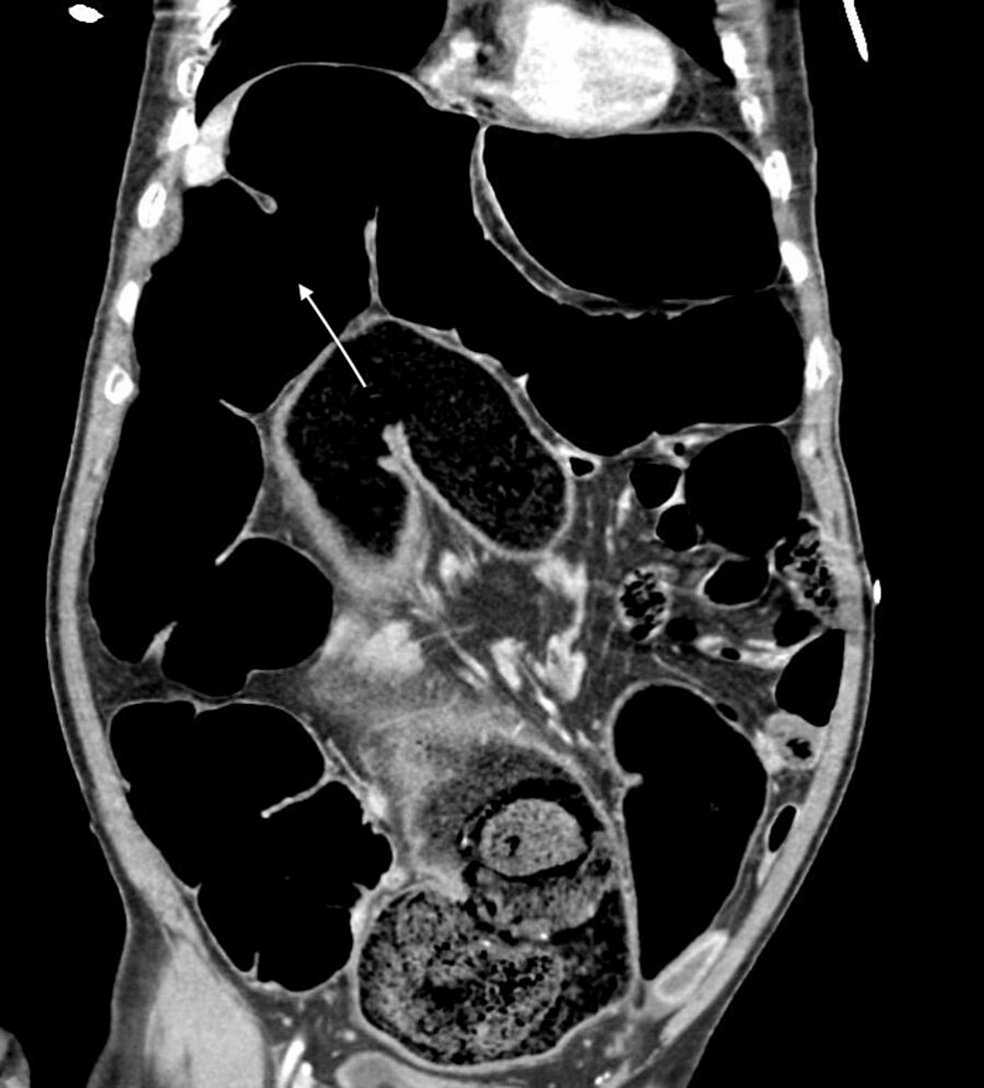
CT image showing dilated large bowel loops
